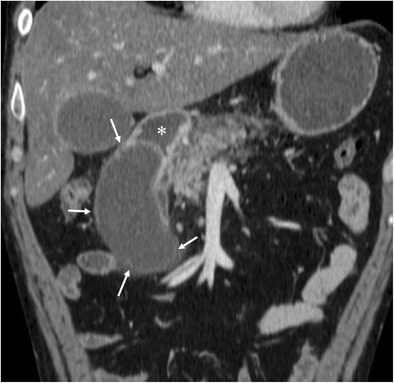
Duodenal duplication cyst. Reformatted coronal contrast-enhanced CT image shows a large well-circumscribed cystic mass (arrows) adjacent to the second duodenal portion. There is no communication with the duodenal lumen (*)
